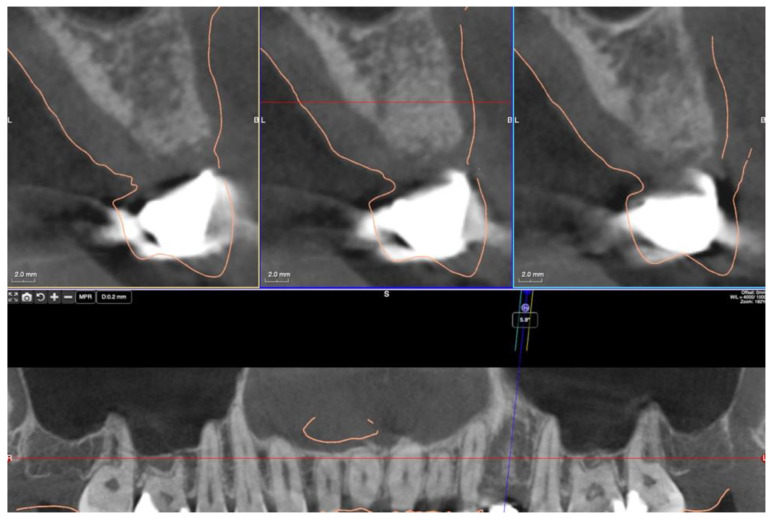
CBCT view six months post-augmentation before implantation in region 24.
